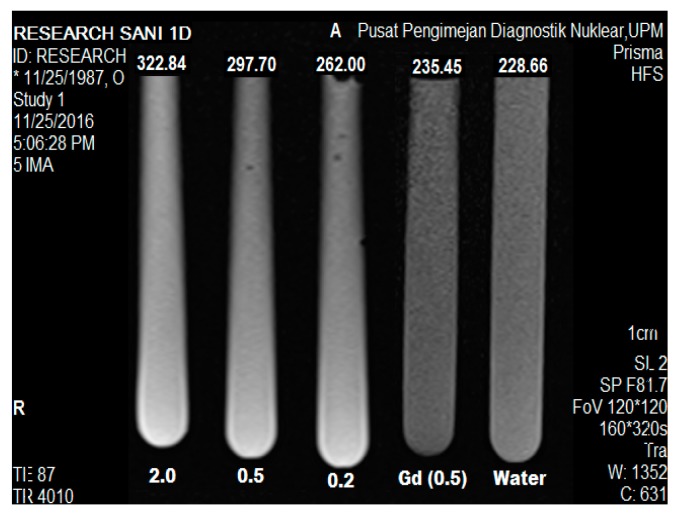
GAGZAu image at different ${\mathrm{Gd}}^{3+}$ concentrations (2.0, 0.5, 0.2), Gd (0.5) *w*/*v* and water taken from magnetic resonance imaging MRI Prisma 3-Tesla machine.
